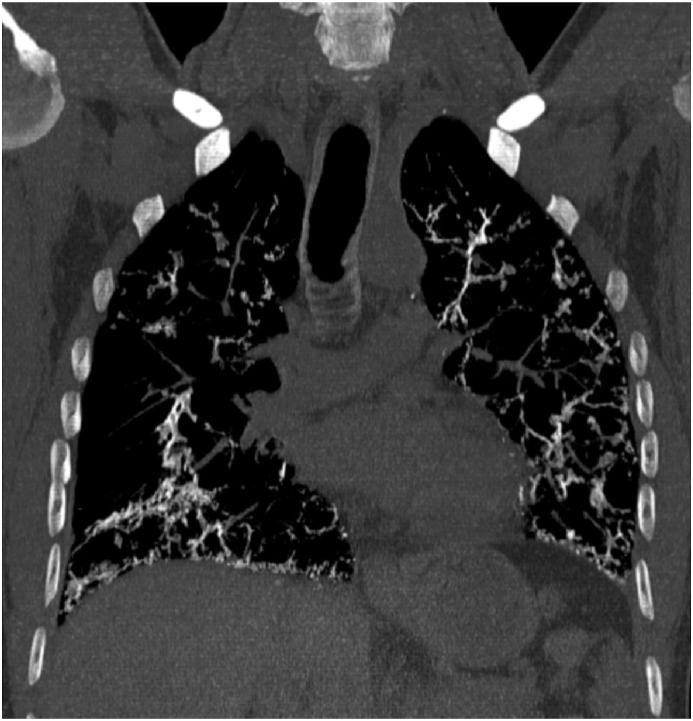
Coronal cut of a high resolution computed tomographic scan of the chest on inspiration using maximal intensity projection using bone windowing demonstrating the diffuse parenchymal calcifications throughout all lobes of the bilateral lungs.
